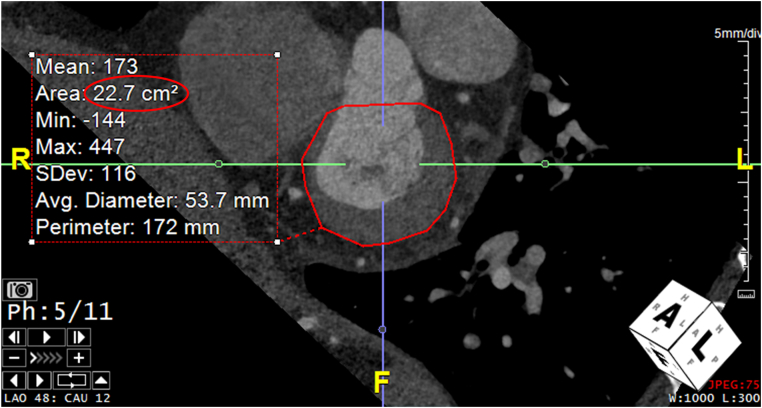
Measurement of LV Short Axis Area using Cardiac CT The volume of pumped blood generated by the AVPD (VAVPD) was calculated as the LV short-axis area multiplied by the AVPD height. As demonstrated by Carlsson et al^2^ and validated for cardiac MRI, the area multiplied by the AVPD cannot be the short-axis area at the mitral annulus but rather must be the largest epicardial short-axis area of the LV as it is constant throughout the cardiac cycle. LV = left ventricular; other abbreviations as in Figure 1.
